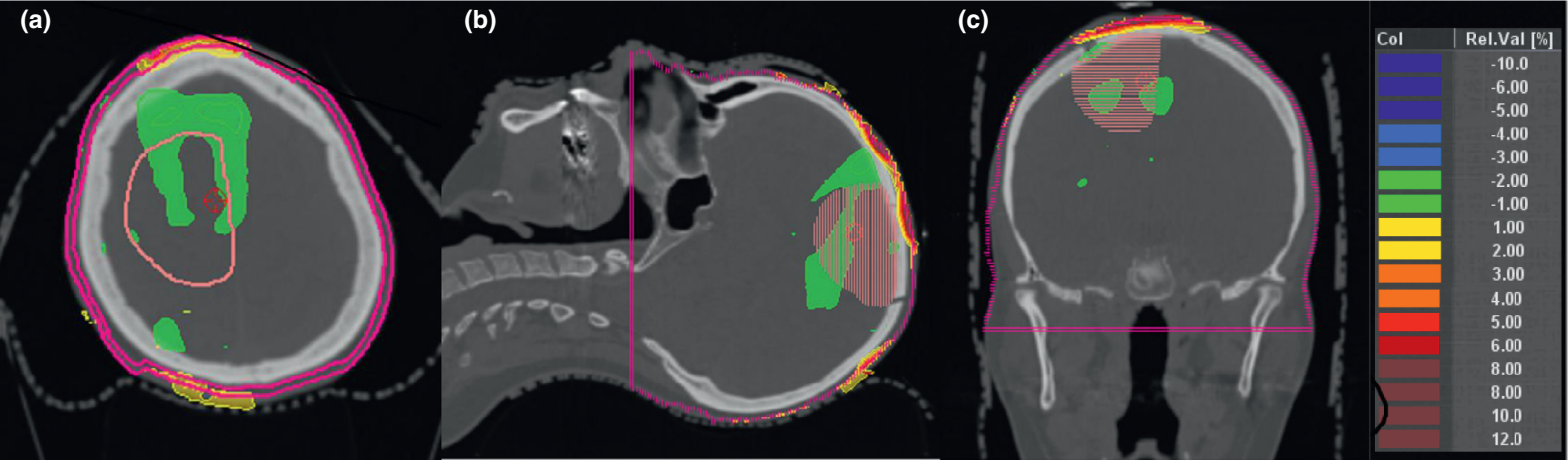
(a–c) Depiction of dose buildup in the superficial scalp layer (2 mm shell contour): Highlighting dose difference plots of accumulated dose distributions without and with TTField electrodes which were fixed at varying positions around a center position during the course of the treatment. Delineated clinical target volume (right frontoparietal), (a) right: axial computed tomography; (b) center: sagittal reconstruction; (c) left: coronar reconstruction. Dose differences are expressed as percentages of the prescribed dose
